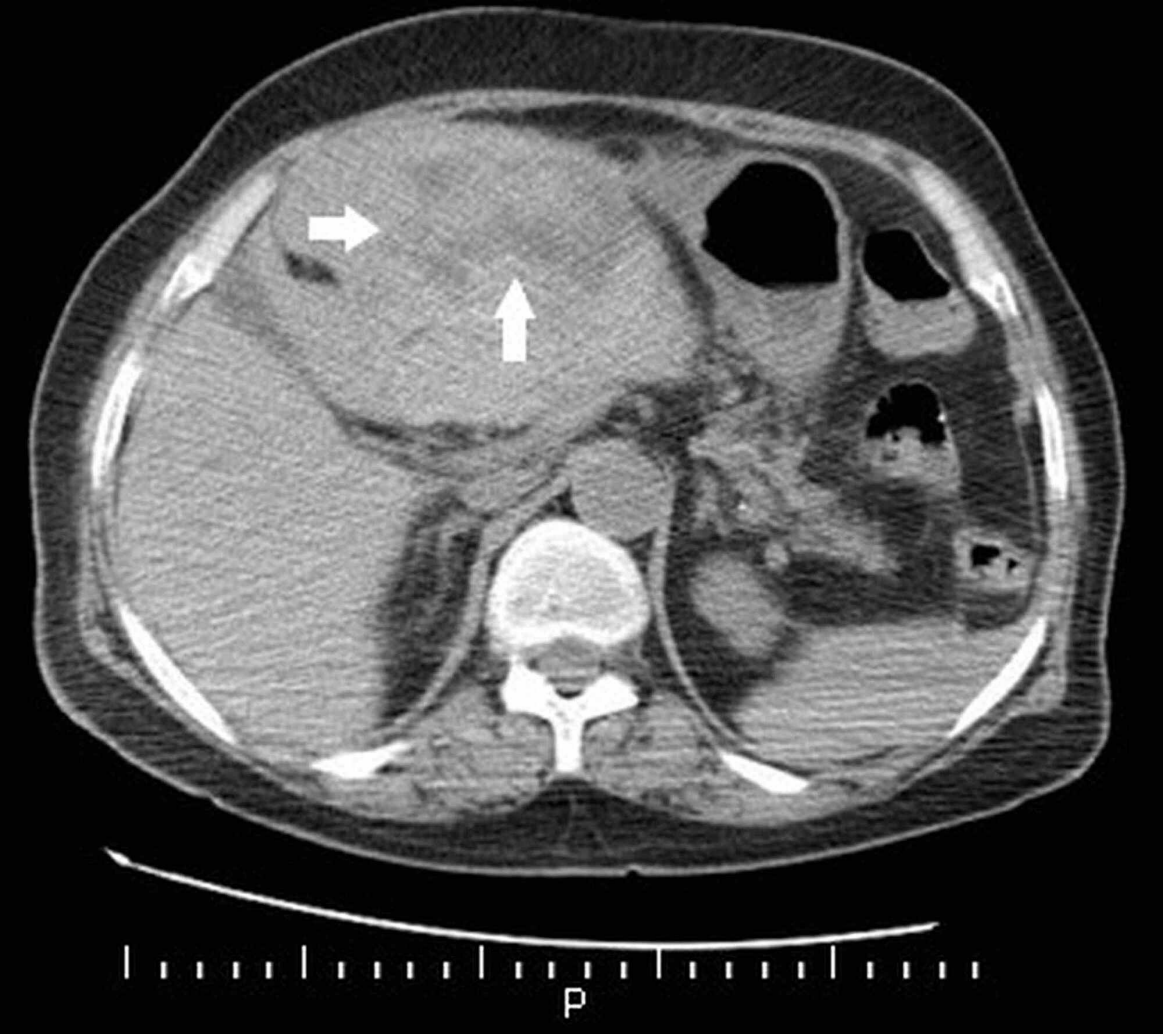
Abdomen computed tomography image: intraparenchymal hemorrhage-hematoma area in the left liver lobe with heterogeneous character around 8 cm (white arrows)
